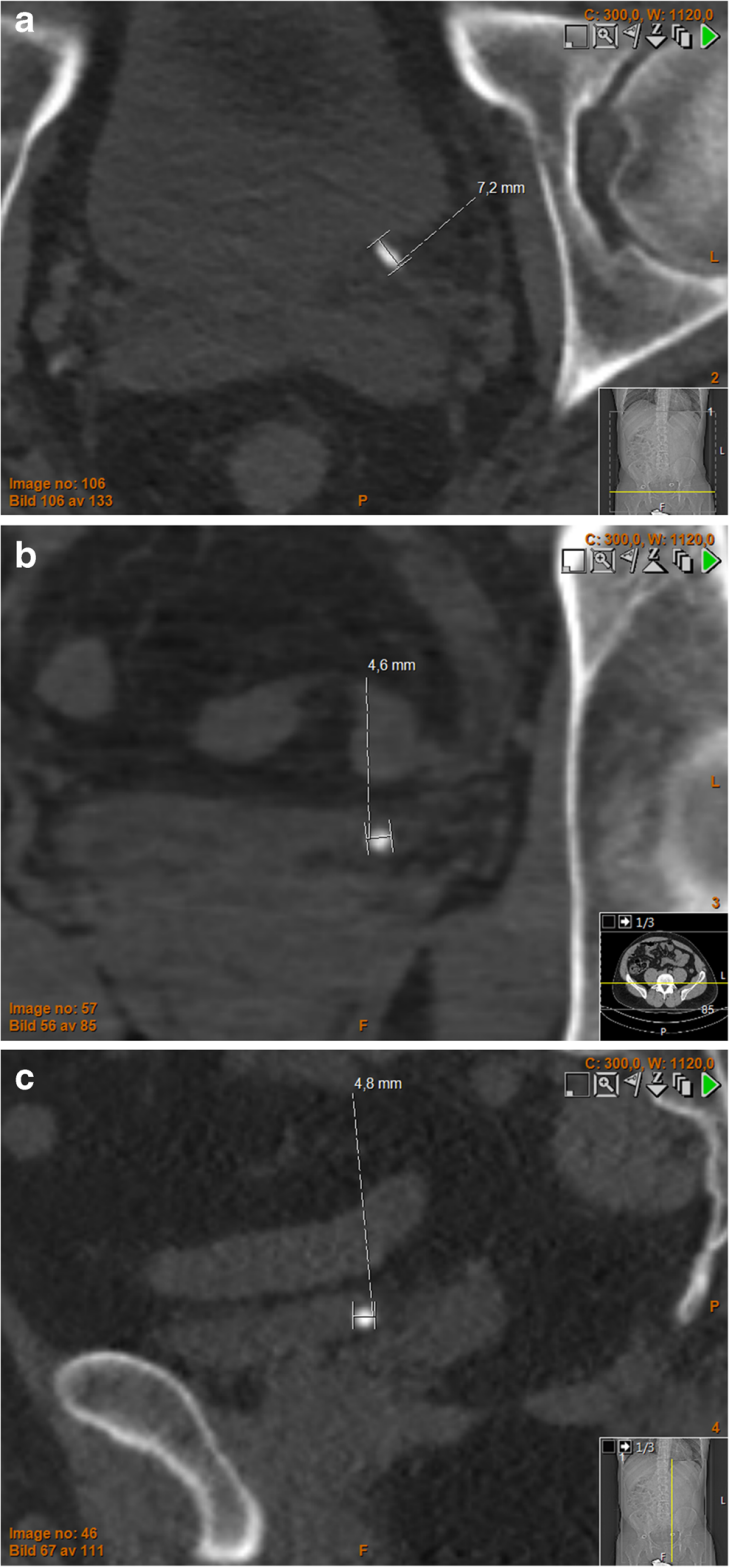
Distal ureteral stone: (a) axial, (b) coronal, (c) sagittal. Non-enhanced computed tomography of the urinary tract, window settings L300/W1120, showing a distal ureteral stone in a 39-year-old male. In each of the three reformats the largest diameter is measured. Stone length = the largest of these measurements (max[ax, cor, sag]). Stone width = the smallest of these measurements (min[ax, cor, sag]). Here the stone length = 7.2 mm and stone width = 4.6 mm
